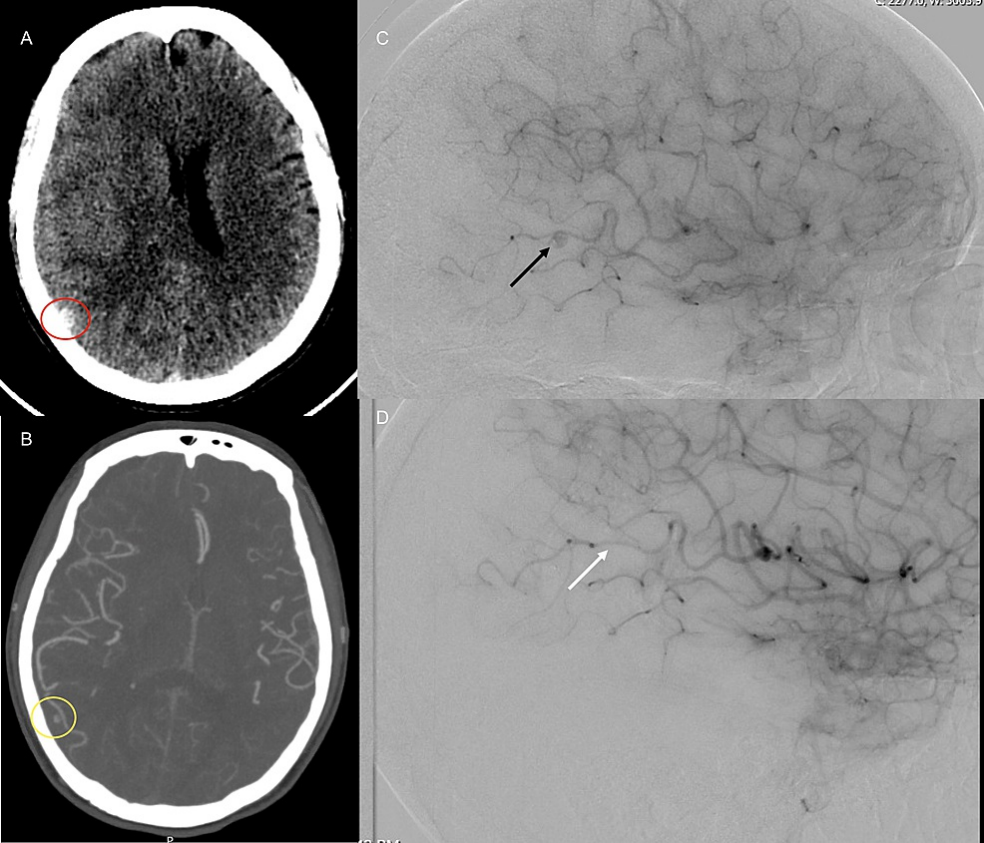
Diagnostic imaging of the head (A) CT head without contrast: Red circle - Right-sided subdural hematoma with mild mass effect. (B) CTA head: Yellow circle - Aneurysm within the right parieto-occipital region. (C) Diagnostic cerebral angiogram: Black arrow - Inferolaterally projecting aneurysm arising from the mid aspect of an M4 right middle cerebral artery branch, specifically the angular artery measuring 3.6 x 3.9 mm. (D) Diagnostic cerebral angiogram (post-surgery): White Arrow – Aneurysm is no longer visualized status post craniotomy and evacuation of subdural hematoma.
